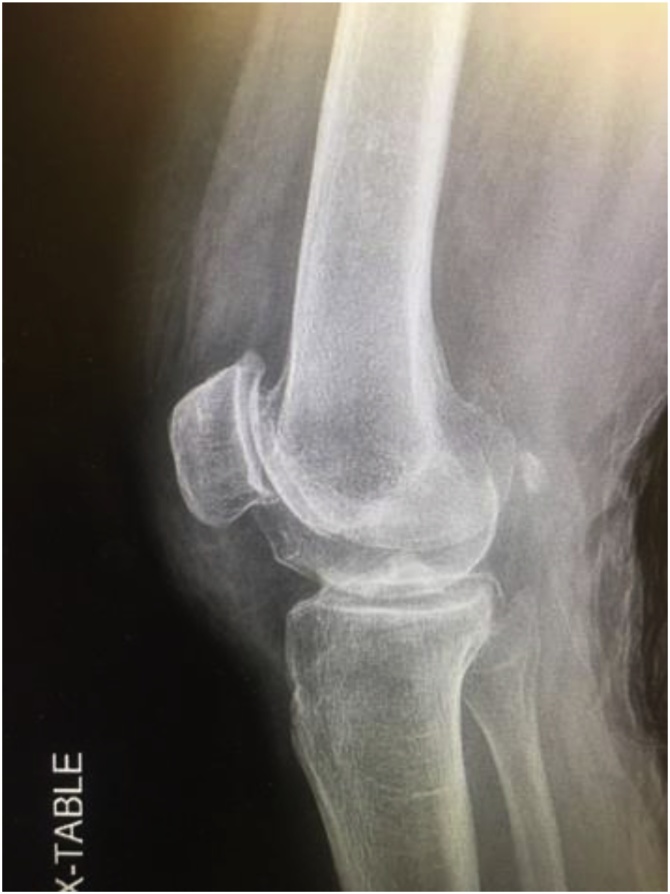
Left Knee Lateral Radiograph (Post Reduction).
